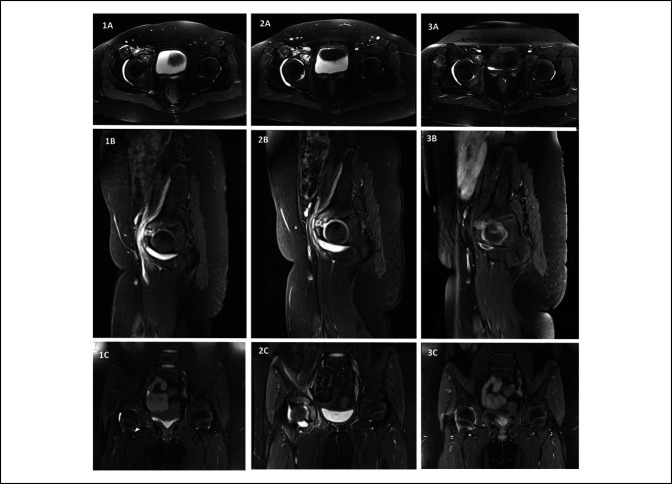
Radiograph showing the T2-weighted pelvic MRI images (A axial, B sagittal, and C coronal) of the patient (1) at the time of admission, (2) immediately preoperatively, and (3) 2 months postoperatively.
